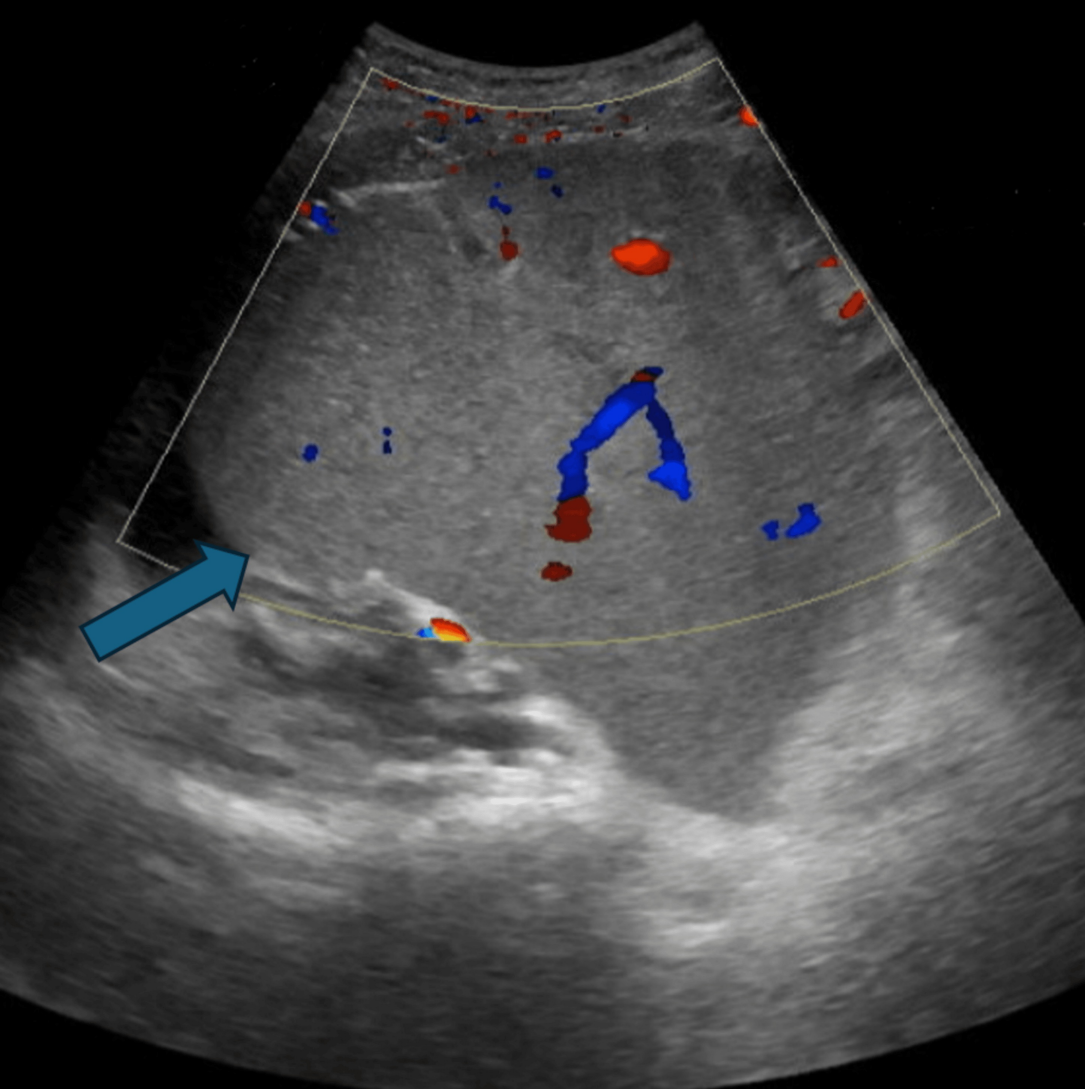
Ultrasound showed enlarged spleen located in the right lower abdomen and pelvis in the subumbilical region, with color doppler flow seen in the splenic hilum and parenchyma Blue arrow: spleen
